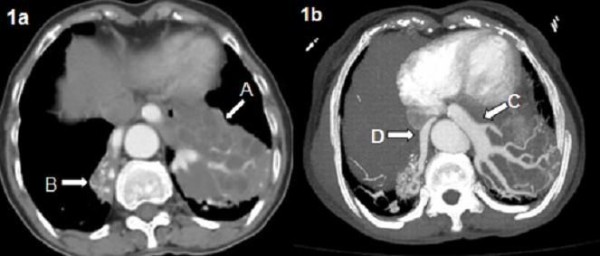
(a) On chest CT transverse section aberrant lung tissue in the posterobasal left lung (*arrow A*) and posterobasal right lung (*arrow B*) are seen. (b) Aberrant arteries are seen leading to aberrant tissue on the left (*arrow C*), and the right (*arrow D*).
